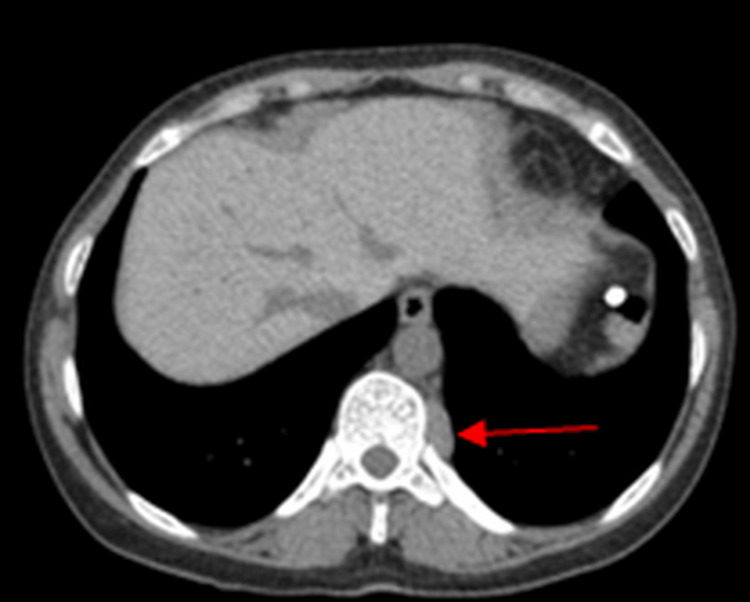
Computed tomography (CT) image from 2017 demonstrating an increase in the size of the paravertebral lesion (arrow).
